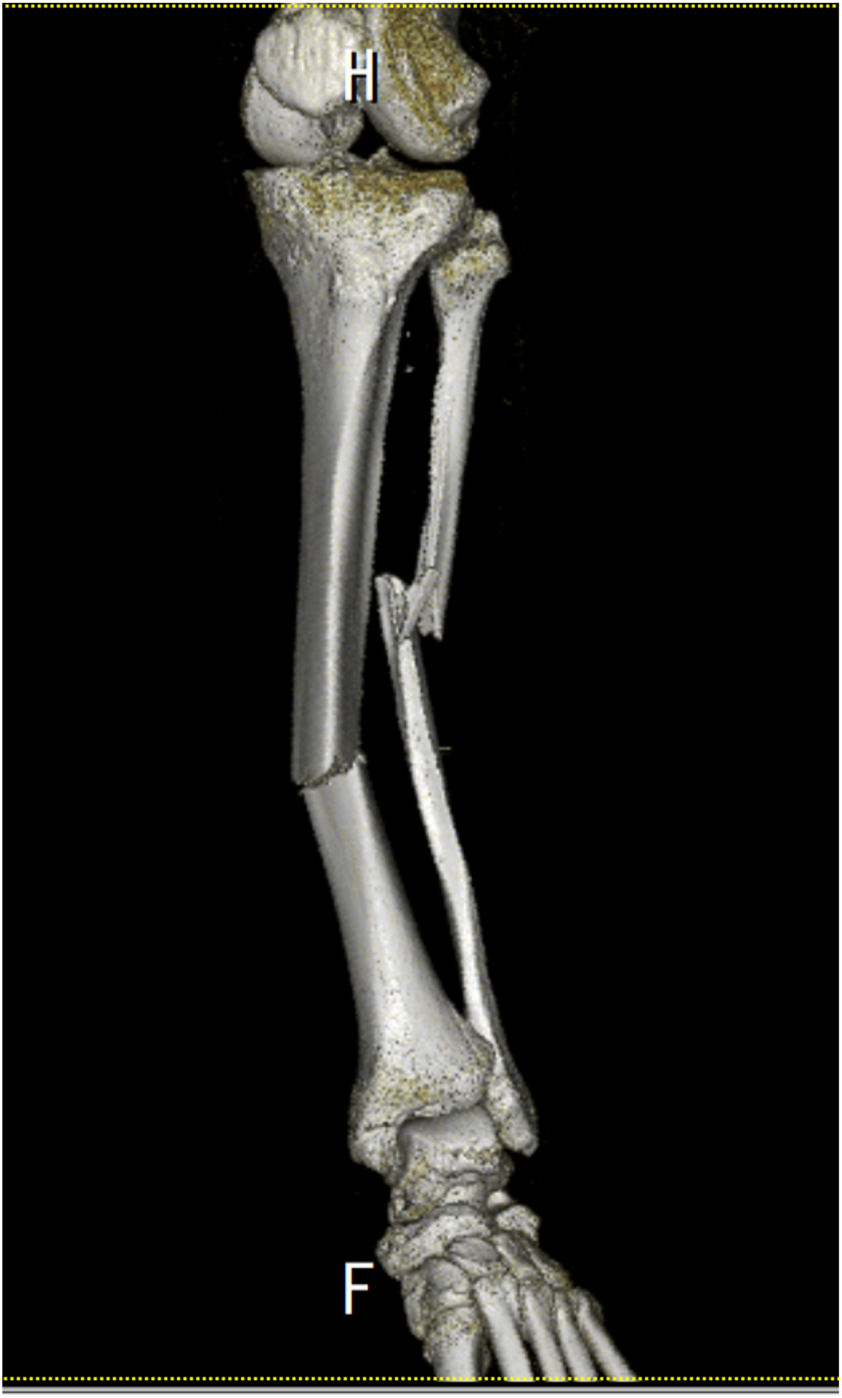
Three‐dimensional volume‐rendered reconstruction of CT images shows the diaphyseal fracture of the left tibia and fibula
